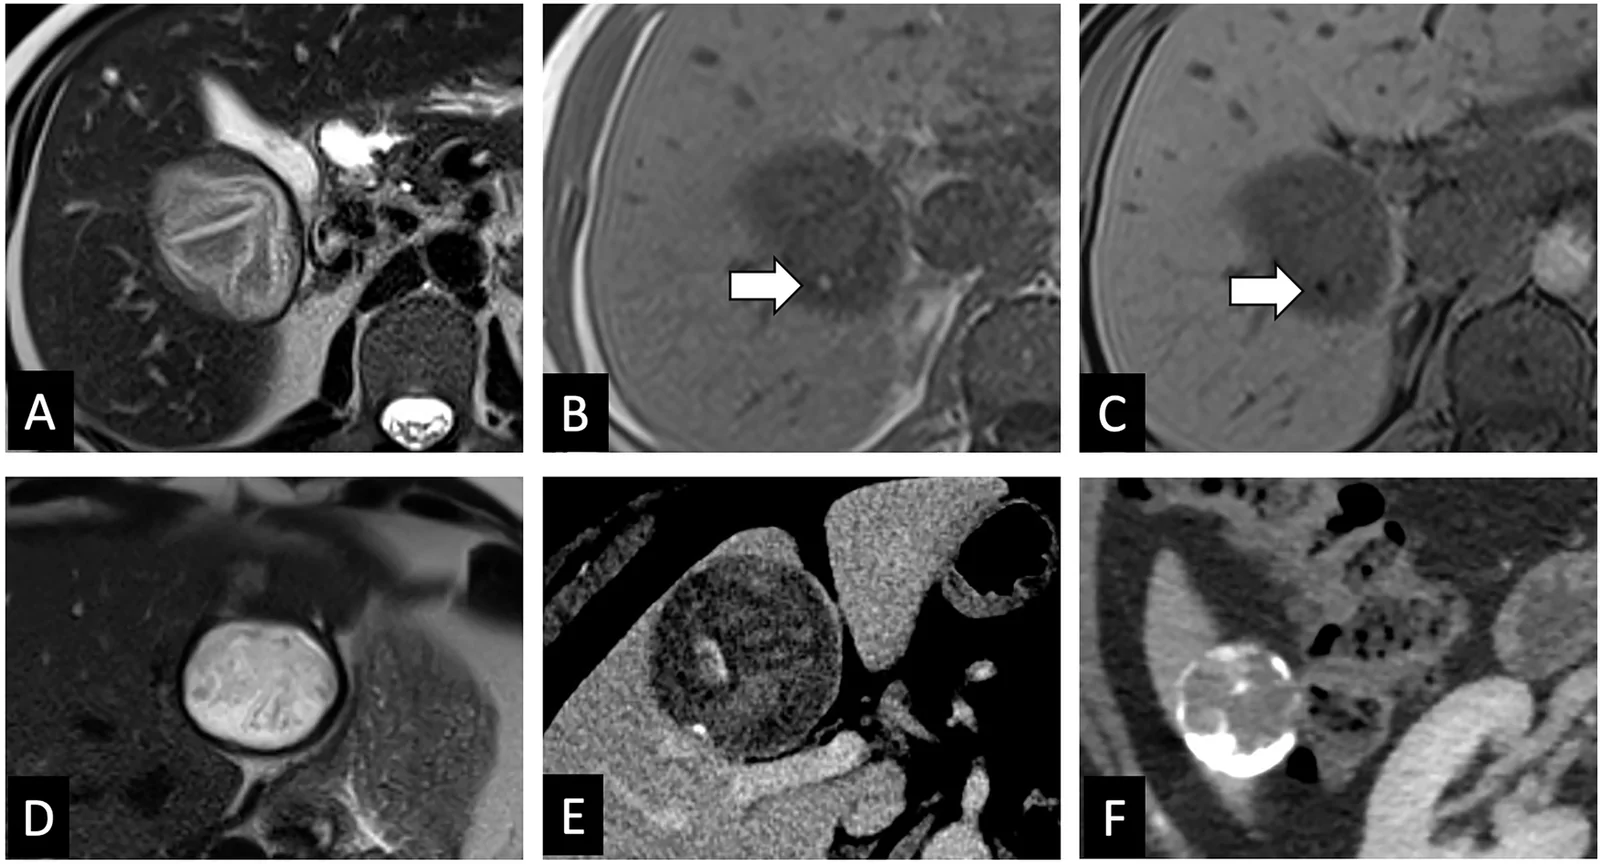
Example of main MRI features associated with parasitic activity. A Matrix flip-flop sign in T2-weighted imaging showing an inverted signal of internal membranes in hypersignal and matrix in hyposignal. B Presence of fatty hypersignal spot within the CE cyst in T1-weighted imaging in phase, in hypersignal (arrow). C Presence of fatty hypersignal spot within the CE cyst in T1-weighted imaging in opposed-phase, in hyposignal (arrow). D Inactive cystic echinococcosis lesion constituted by non-fluid hypersignal T2-weighted. E Cystic echinococcosis cyst with matrix calcifications. F Cystic echinococcosis cyst with internal membranes calcifications
